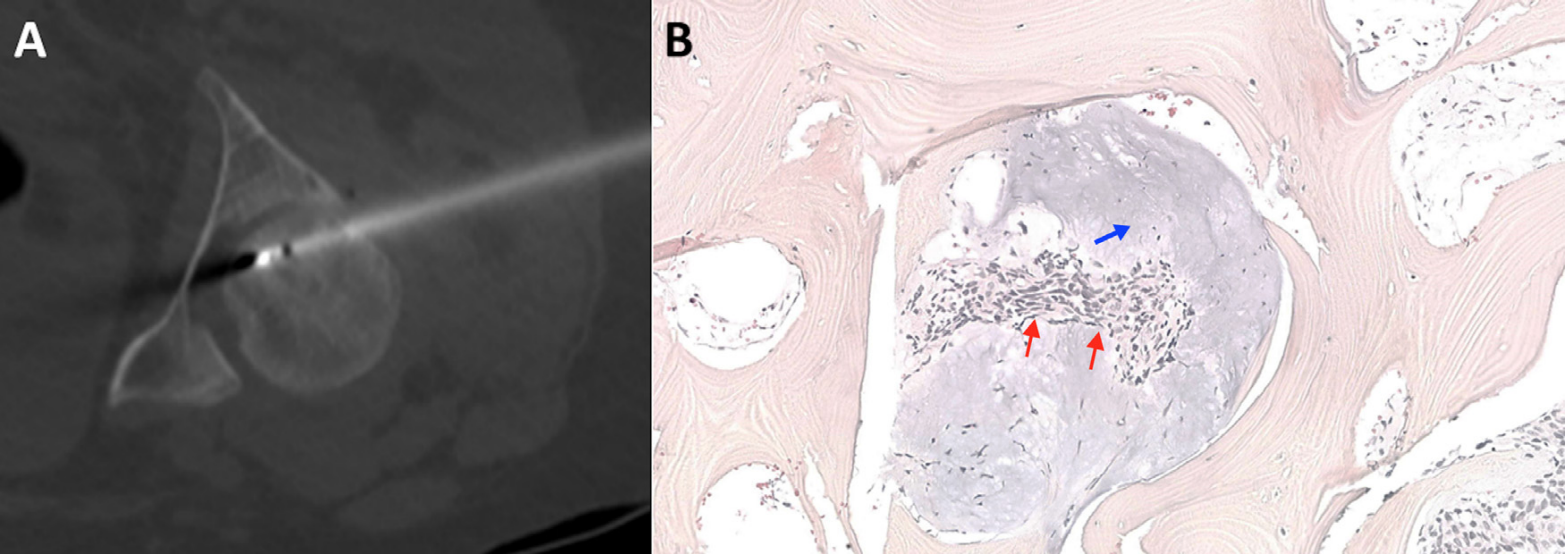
(A) Axial view of the CT during RFA showing the needle in the center of the lesion. (B) High power view showing bland spindled cells (*red arrows*) and basophilic myxoid matrix (*blue arrow*), filling the osteomedullary spaces.
